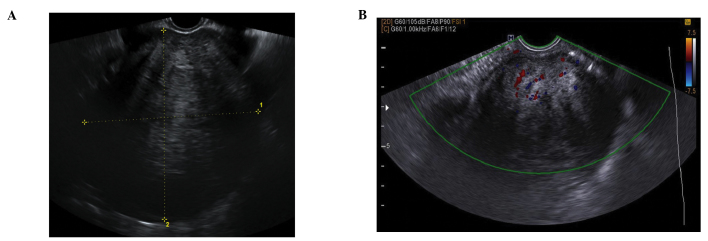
Typical round regular ovarian fibroma with (A) acoustic shadows and (B) minimal peripheral vascularity on color Doppler.
